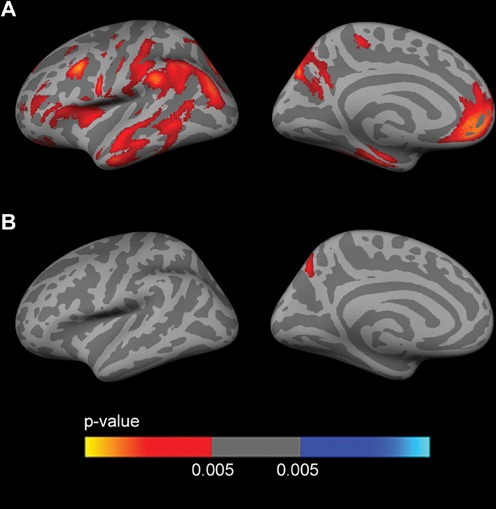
Sex-stratified FDG analyses Analysis between *APOE4* carriers and *APOE4* non-carriers (*p* < 0.005 uncorrected) in A. females and B. males, co-varied for age and years of education across the lateral and medial views of the cerebral cortex. As shown, female *APOE4* carriers showed widespread clusters of decreased metabolism with respect to female *APOE4* non-carriers (Fig. 2A), whereas male *APOE4* carriers only showed an isolated cluster of decreased metabolism (*p* < 0.005) in the precuneus with respect to male non-carriers (Fig. 2B). FDG = fluorodeoxyglucose; *APOE4* = apolipoprotein E ε4 allele.
